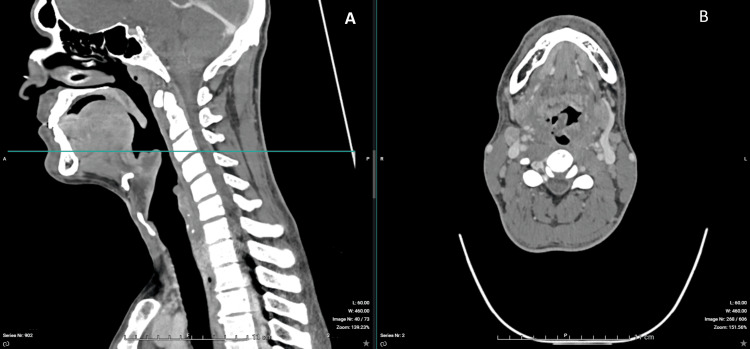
Contrast-enhanced CT imaging of the epiglottis. (A) Sagittal view (left) demonstrating a markedly thickened and edematous epiglottis causing significant mass effect on the airway. The horizontal line indicates the level of the axial section. (B) Axial view (right) demonstrating severe narrowing of the supraglottic lumen, consistent with impending airway compromise.
